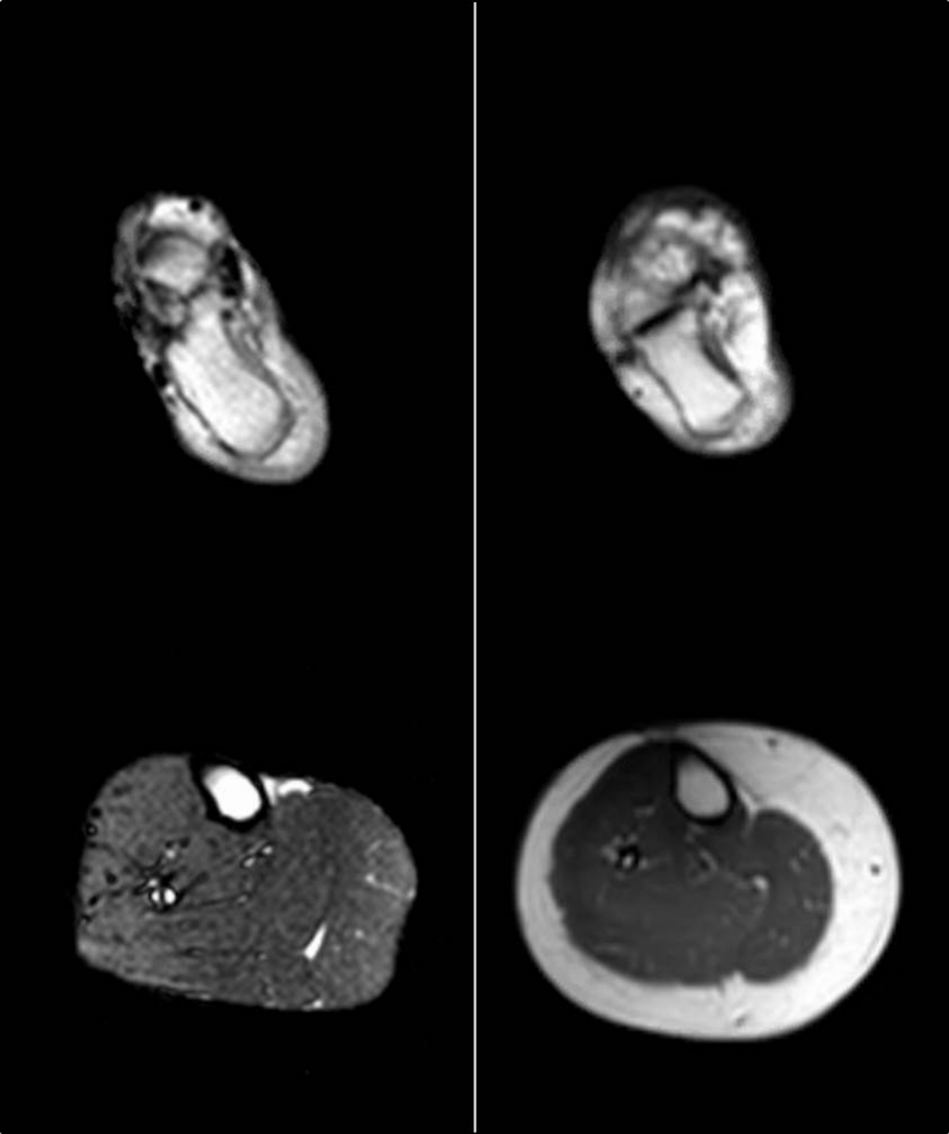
Magnetic resonance (MR) images of a sample patient and a healthy control. MR imaging using as a standard T1 sequence shows the right heel (upper row) and proximal calf (lower row) of a female patient with lipodystrophy (left column, BMI 27 kg/m²) and a female control (right, BMI 31 kg/m²). Written consent for publication of MR images was obtained from both subjects.
